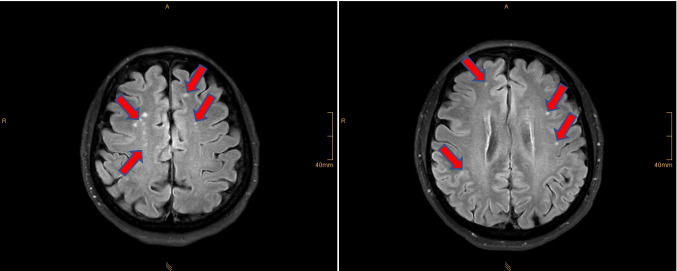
Brain-MRI; the multi-site pattern of lacunar microinfarction zones is clearly visible in the image (red arrows); 3Tesla-MRI was performed at the radiological clinic, University of Bonn, Germany; weight-adjusted intravenous contrast medium administration (CMA, gadolinium) was used
